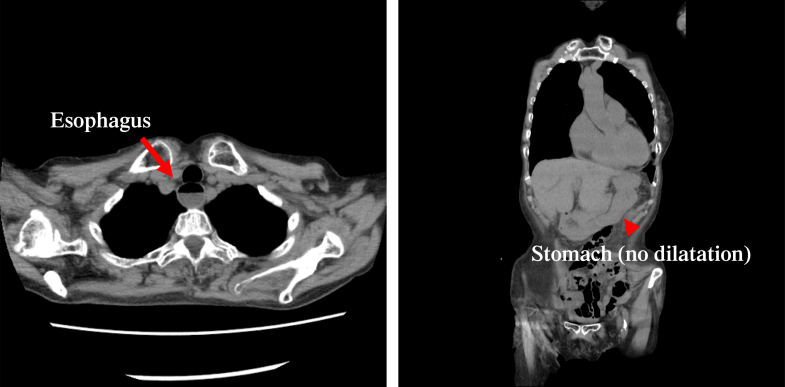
Chest computed tomography findings after ablation. Chest computed tomography images demonstrate marked esophageal dilatation with intraluminal contents, without evidence of gastric dilatation. The red arrows indicate esophageal dilatation at the level of the main bronchus. In contrast, the stomach (indicated by the red arrowhead) shows no evidence of gastric dilatation.
